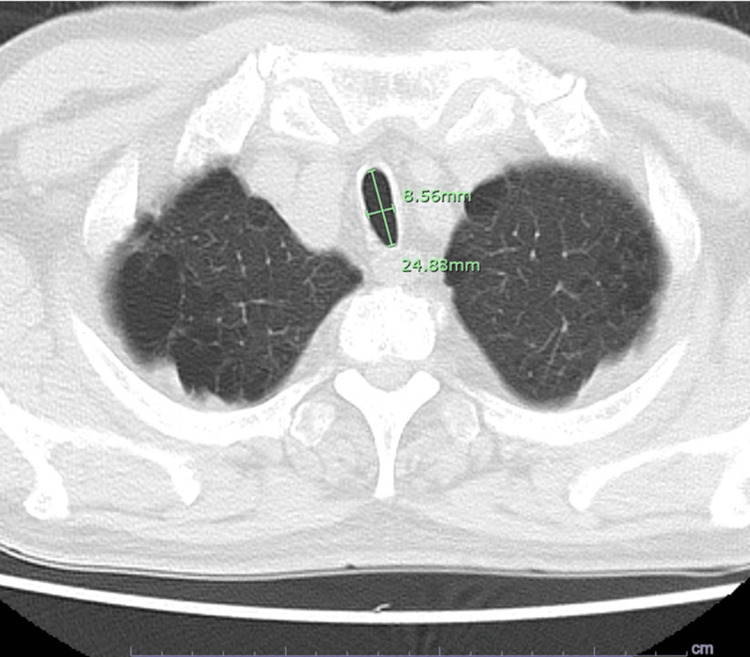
Axial chest computed tomography demonstrating saber-sheath trachea. Axial chest computed tomography image demonstrating marked transverse narrowing with relative anteroposterior widening of the trachea, consistent with saber-sheath trachea. At the narrowest segment, the transverse diameter was 8.56 mm and the anteroposterior diameter was 24.8 mm (tracheal index, 0.34).
